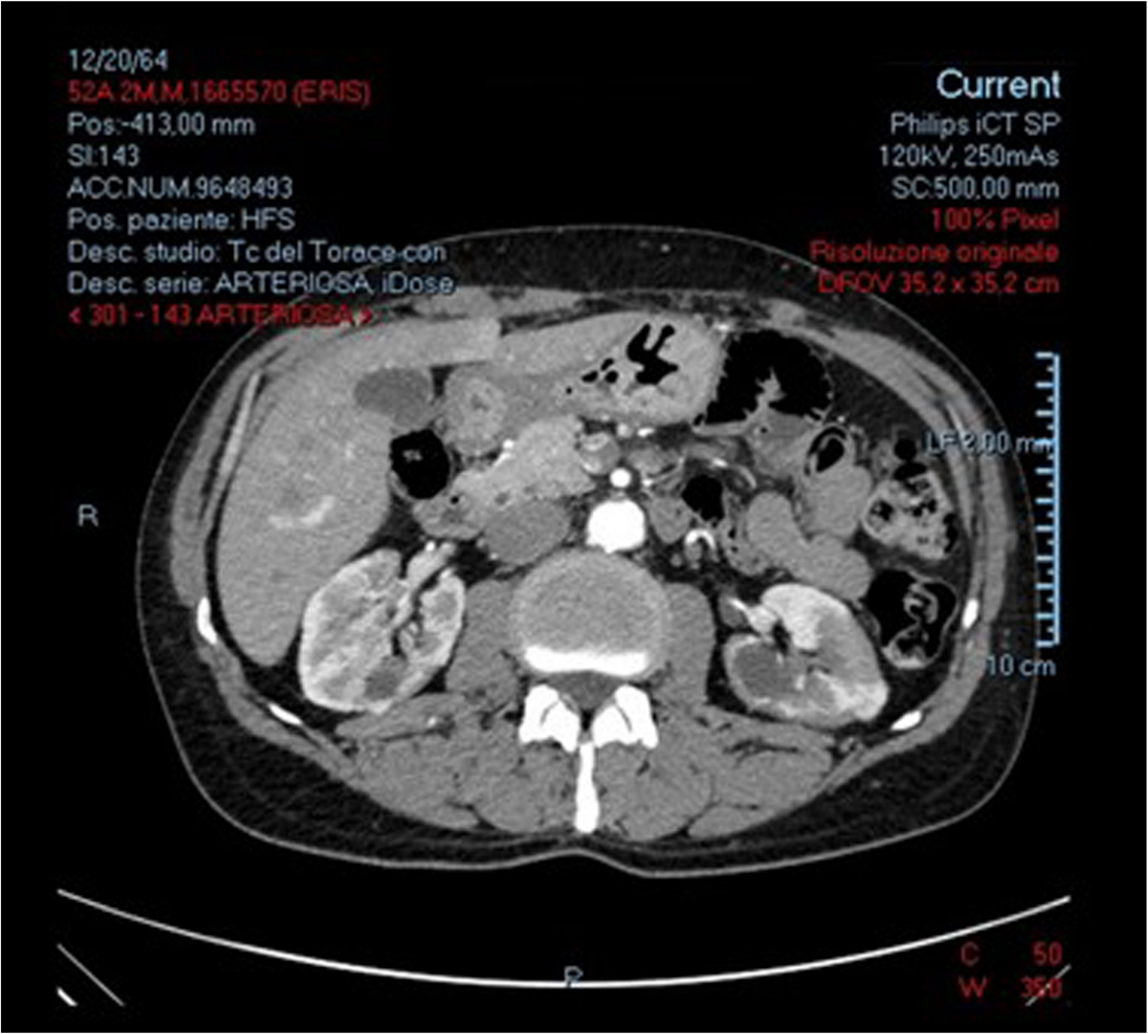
Bilateral renal low density lesions on enhanced computed tomography (CT)
